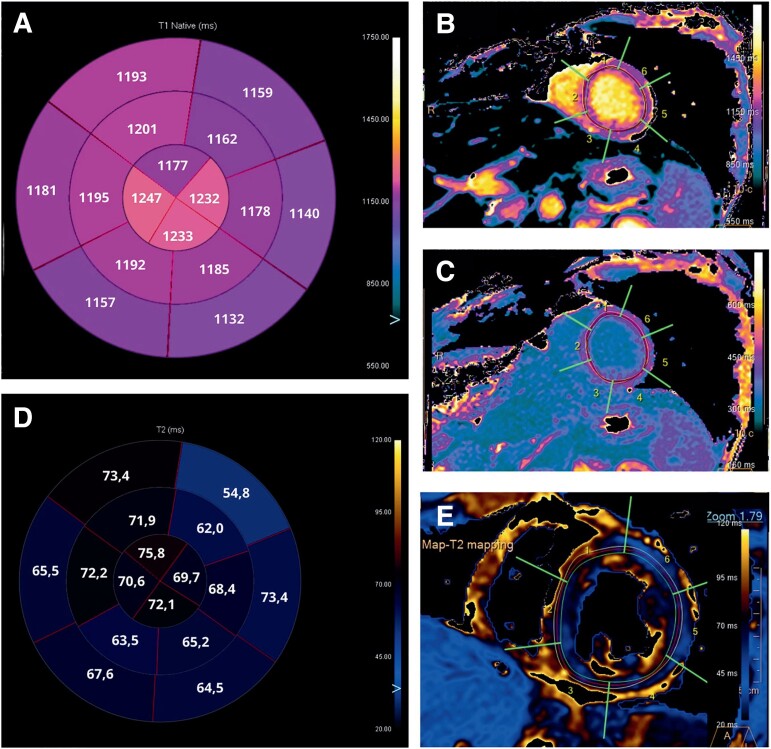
Cardiac magnetic resonance images. (*A*) Bullseye plot showing the native T1 times. Segmentation was performed according to the AHA 16-segment model. (*B* and *C*) Native T1 times and T1-enhanced were increased in all segments, especially in mid and apical segments. (*D*) Bullseye plot showing the native T2 times. (*E*) Native T2 times were increased in all segments, especially in mid and apical segments.
